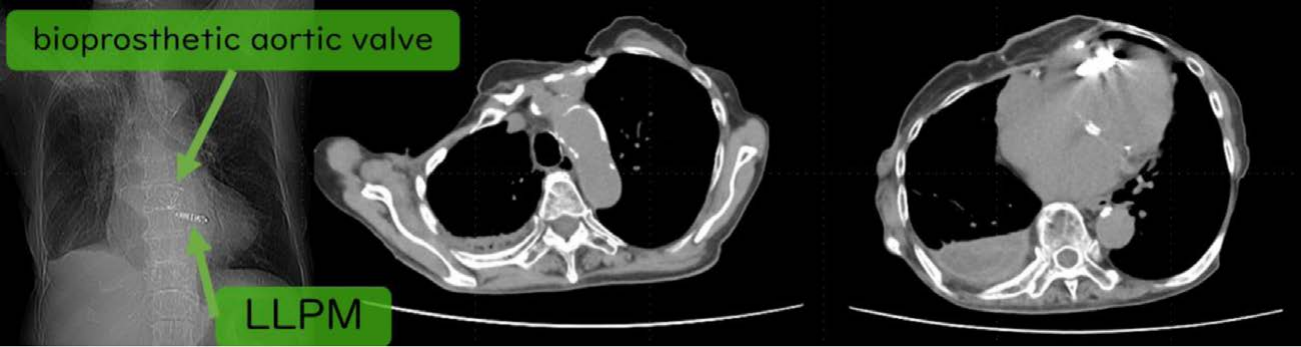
Computed tomography images showing no identifiable pacemaker or lead wires in the scout view or transverse images of the left anterior chest. A leadless pacemaker implanted in the patient’s right ventricle. LLPM = leadless pacemaker.
